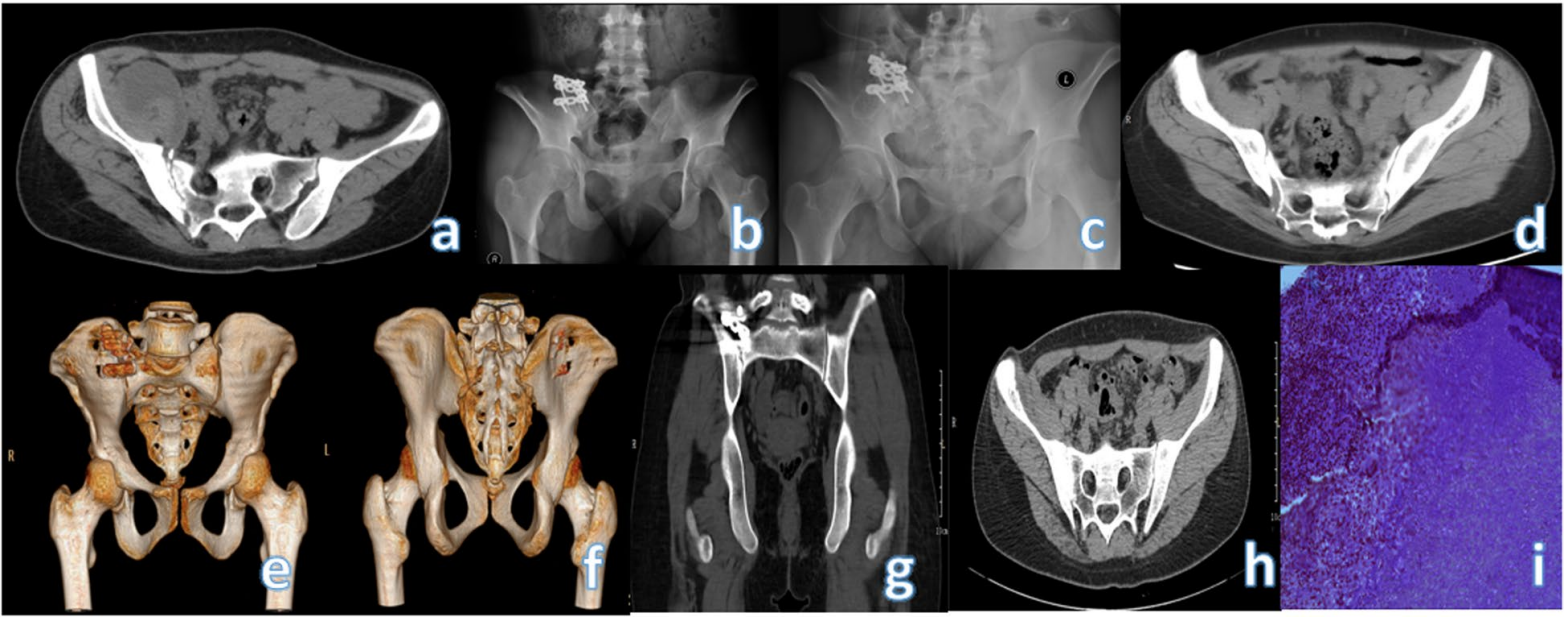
Preoperative CT, X-ray at 3 months after operation, X-ray at 6 months after operation, and CT at 12 months after operation of NO.6. a The right sacroiliac joint space is widened, the articular surface is not smooth and tidy, the band-like bone density is increased, and the articular surface of the iliac flank is destroyed by insects. b The implanted autologous ilium did not see obvious bone resorption occurred 3 months after surgery. c Part of the bone in the operation area can be seen fused 6 months after the operation, and the bone density in the operation area is not uniform. d-h Three-dimensional reconstruction imaging of the sacroiliac joint showed that a relatively stable arthrodesis had been achieved 12 months postoperatively
